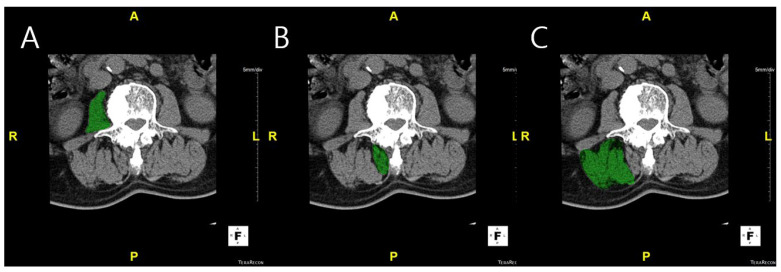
Axial CT images showing ROI of paraspinal muscles using Aquarius iNtuition Viewer (TeraRecon Inc., Foster City, CA, USA). The muscles highlighted in green indicate the segmented ROI. (A) Psoas muscle. (B) Multifidus muscle. (C) Erector spinae muscle.
